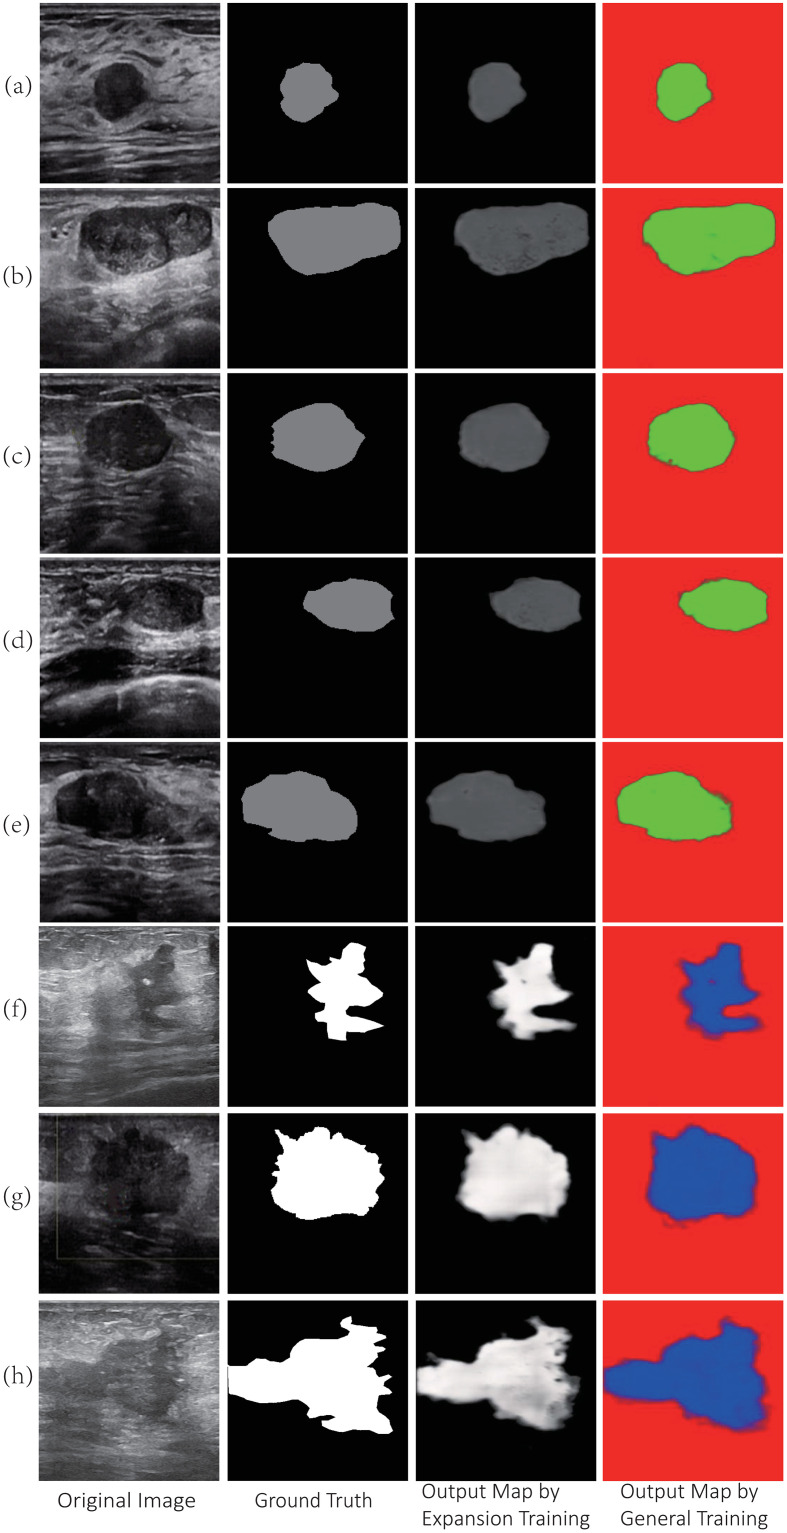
Comparison of test results in breast ultrasound images.
